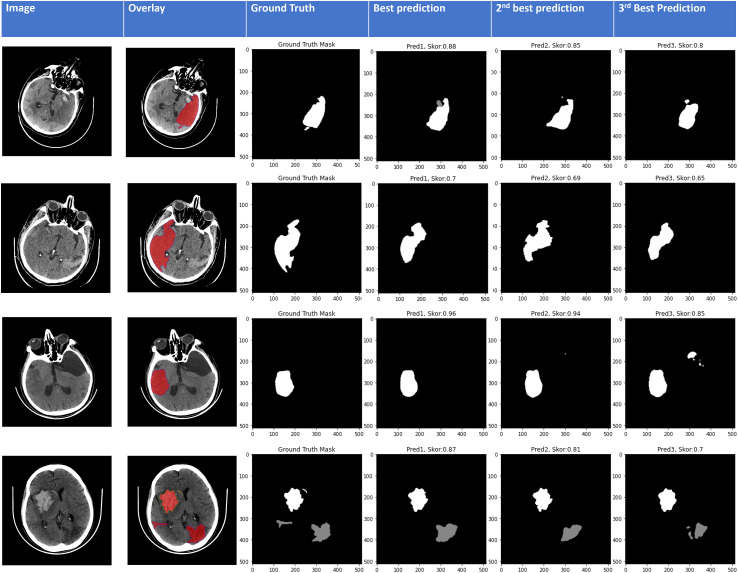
Top 3 IoU scores for 4 images (misleading images). First row CT image consisted of both ischemic core and aneurysm ipsilaterally, second row CT image had both ischemic core and vascular anomaly contralaterally, third row CT image had arachnoid cyst and ischemic core contralaterally, and fourth row CT image had both ischemic cores and hemorrhagic areas. CT, computed tomography.
